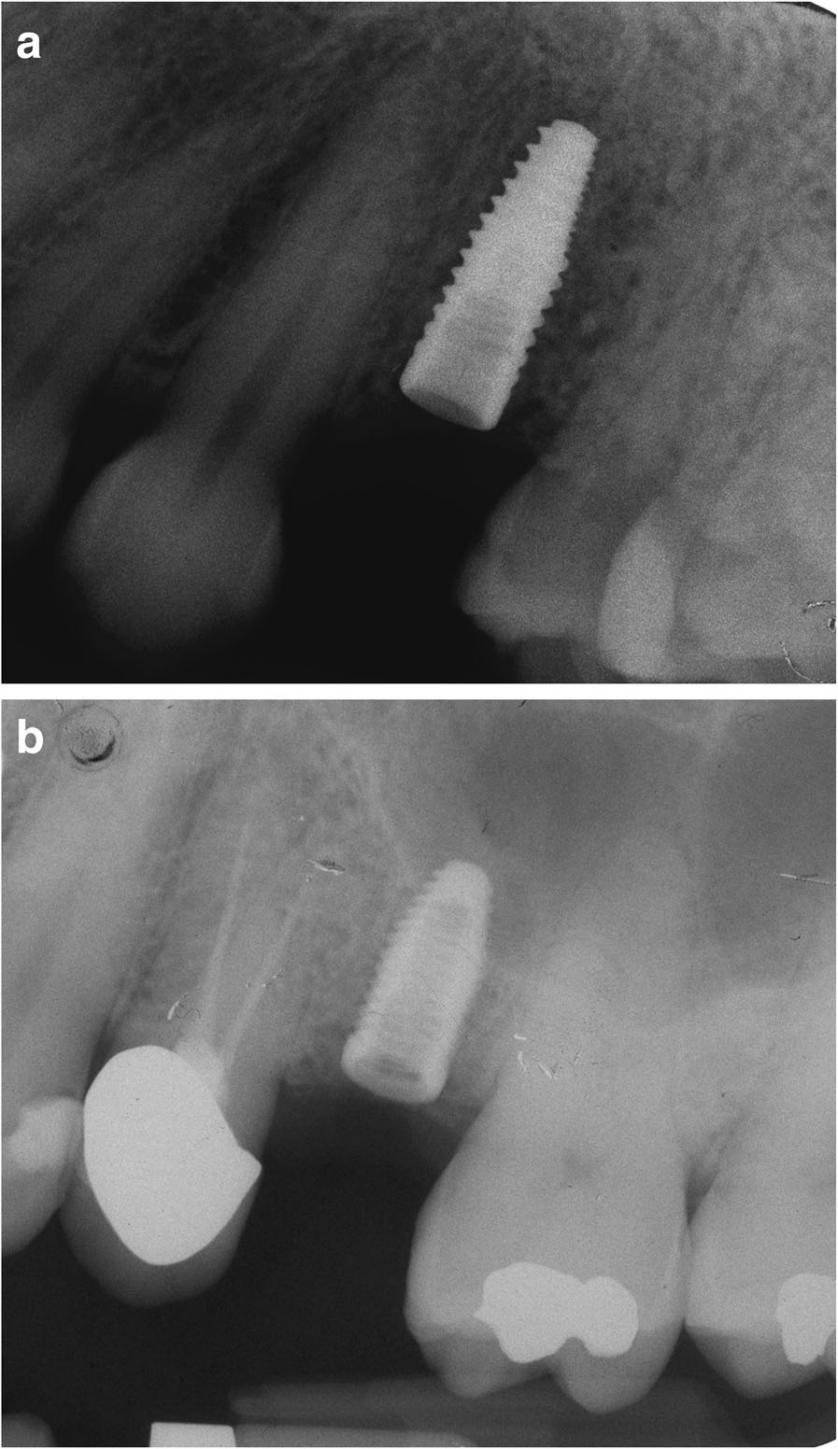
a, b: Postoperative radiographs
